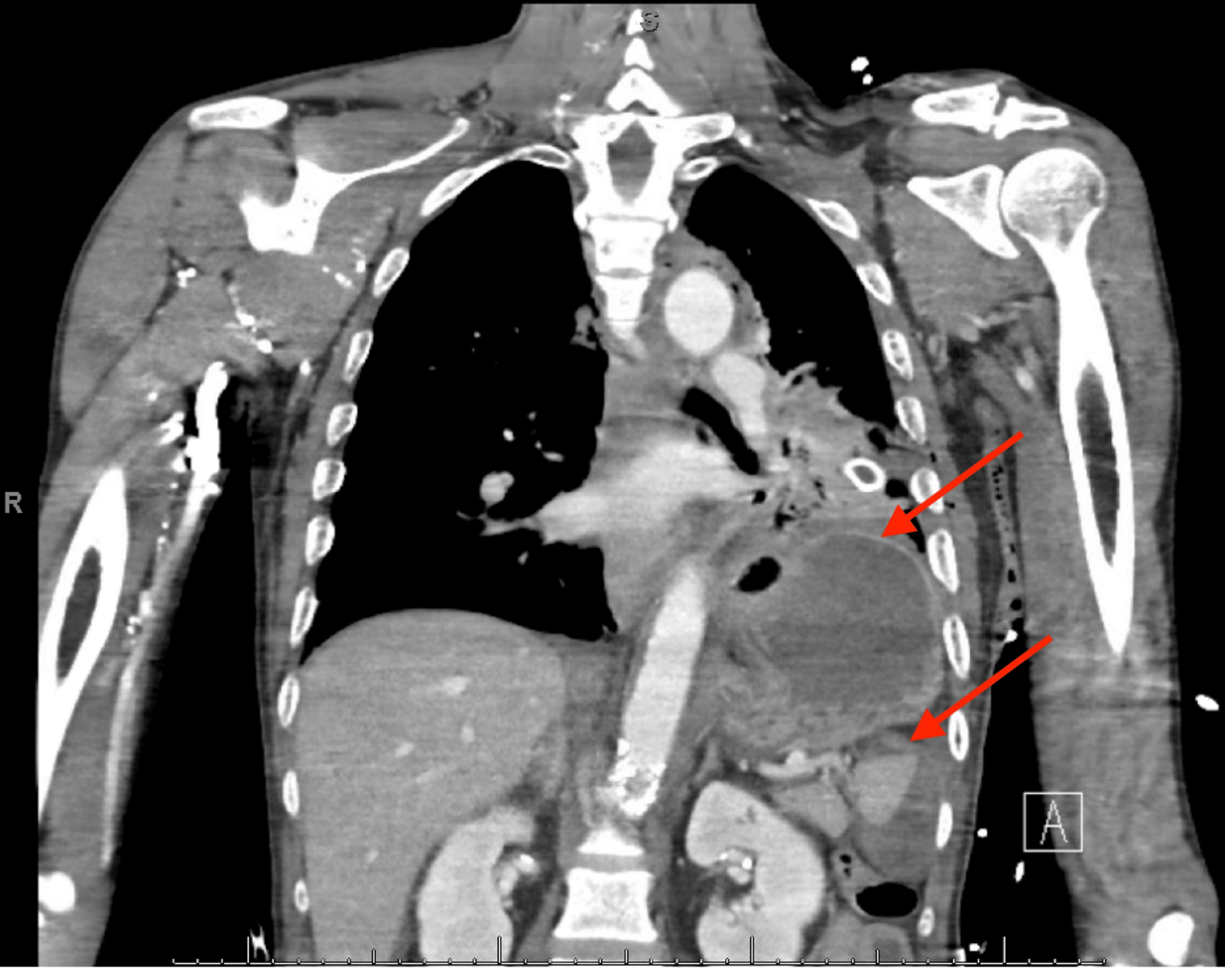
Reconstructed coronal view of chest CT with gastric fundus appreciated within the left pleural space This image shows a traumatic left diaphragmatic hernia measuring 6.9 cm with herniation of the stomach into the thoracic cavity.
